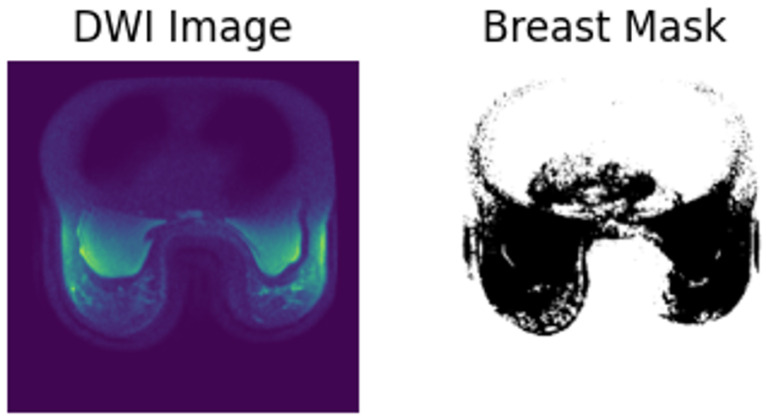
Sample breast mask generated from the DWI image.
